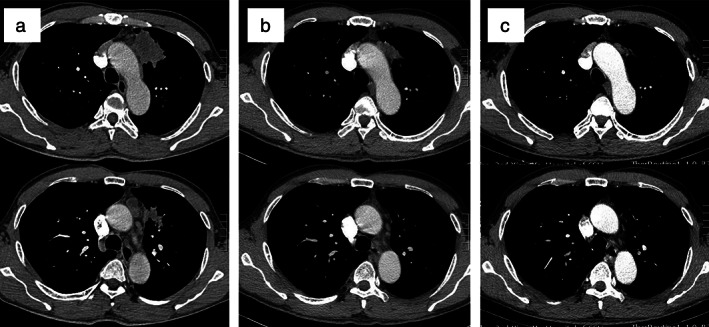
Evaluation by computed tomography (CT) scan. (a) At diagnosis. The upper left lobe mass was considered as the primary tumor while the mediastinal mass was considered as station 5 and 6 lymph‐node metastasis. (b) One month after osimertinib, both the primary tumor and lymph‐node had decreased in size. (c) Three months after osimertinib. The primary tumor had reduced in size remarkably, and the mediastinal mass had disappeared.
